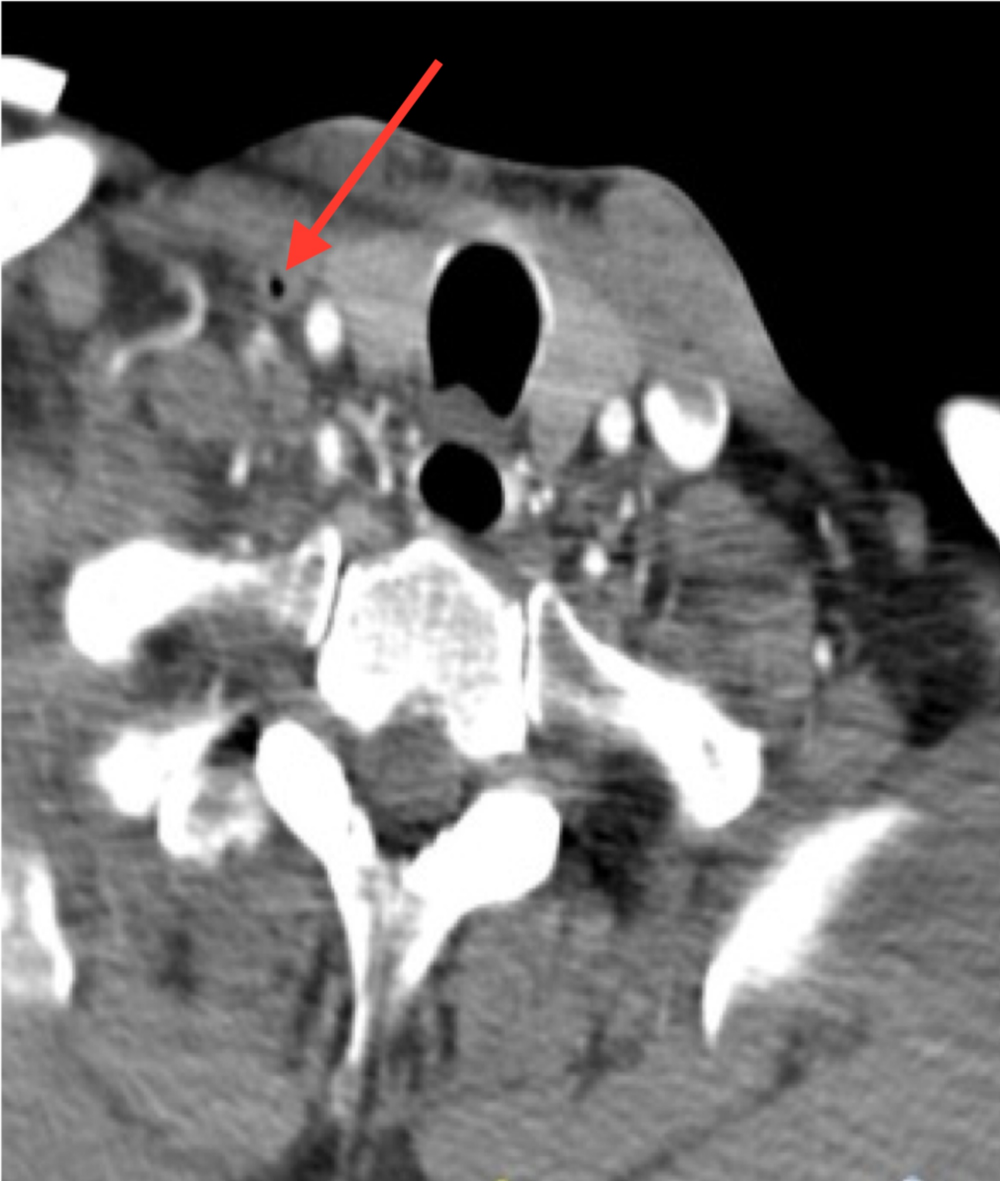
Coronal slice from the computed tomography (CT) angiogram at the level of the trachea with a red arrow showing air in the right internal jugular vein
